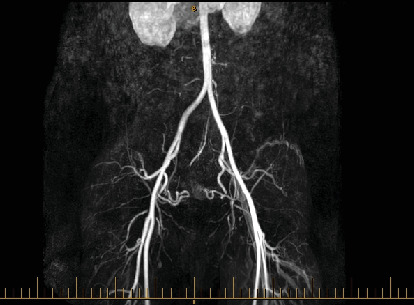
Follow-up angio-MRI demonstrating the regression of the uterine AVM.
